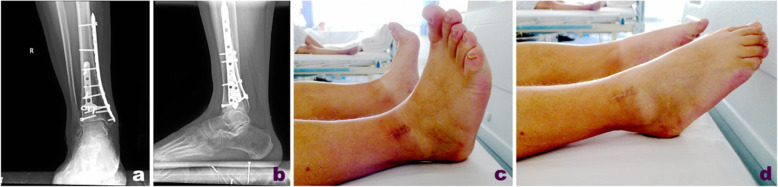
At 48 months follow-up, X-ray reexamination showed that the fracture healed, no loosening or breakage of internal fixation was observed, and function recovered well (a–d)
